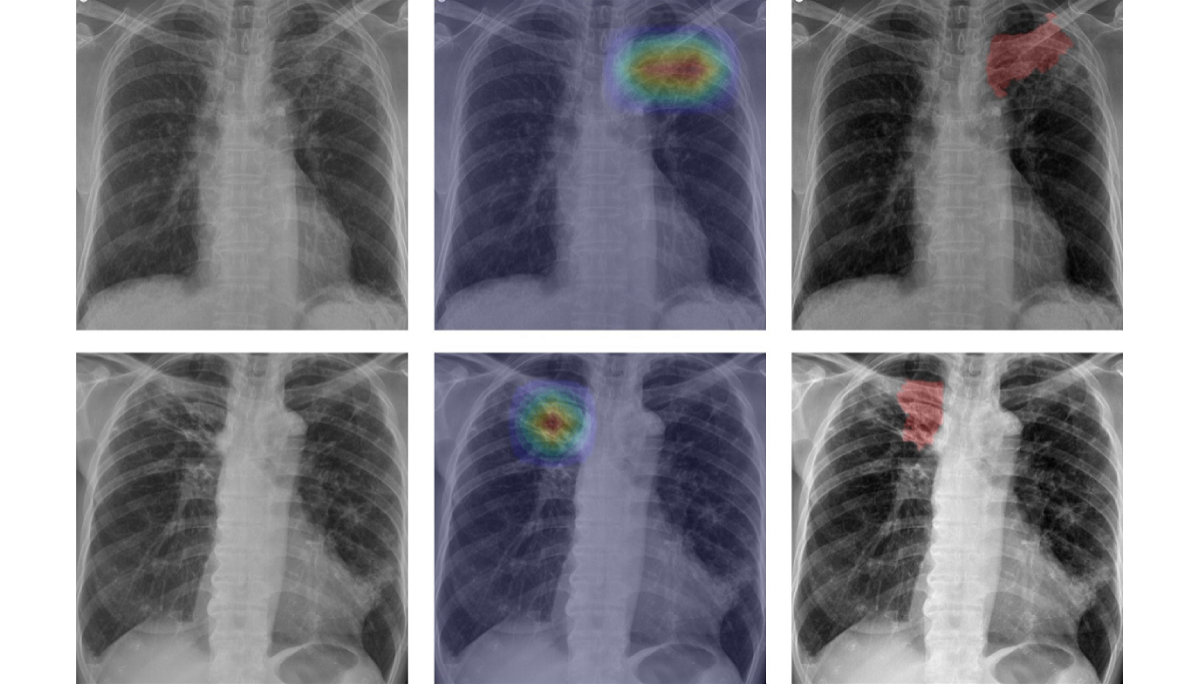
Left: chest radiographs used for the analysis; middle: results of GradCAM; and right: results of LIME. Both the upper and lower panels display chest radiographs that were accurately identified as positive by the model. The analysis in the upper figure focuses on the left lung apex, while the analysis in the lower figure concentrates on the right lung apex, visualizing the areas of frequent tuberculosis occurrences. GradCAM: gradient-weighted class activation mapping; LIME: local interpretable model-agnostic explanations.
